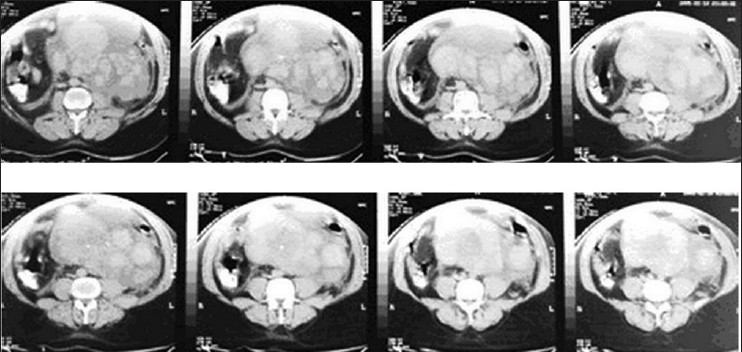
CT scan; multiple cuts show the extent of the blood cyst at different levels
